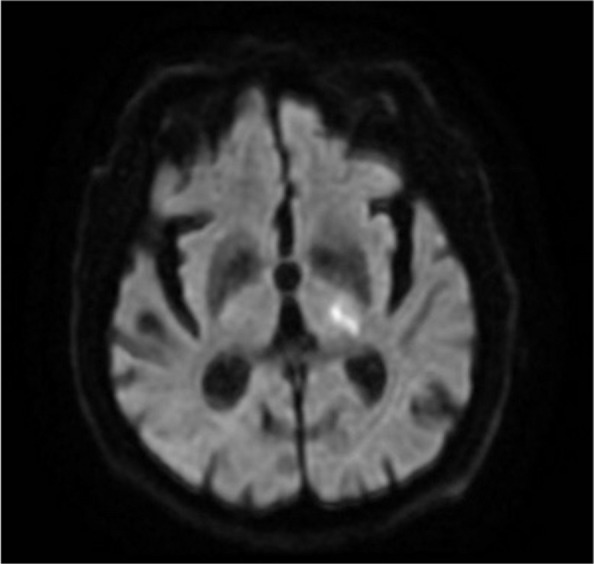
Restricted diffusion in the left thalamus on DWI MRI
